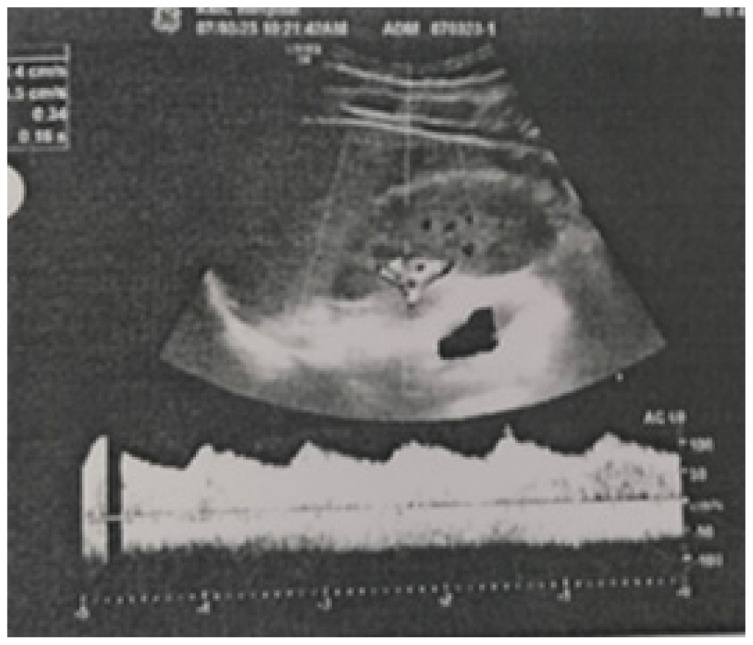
Doppler ultrasound showing Tardus Parvus waveforms.
